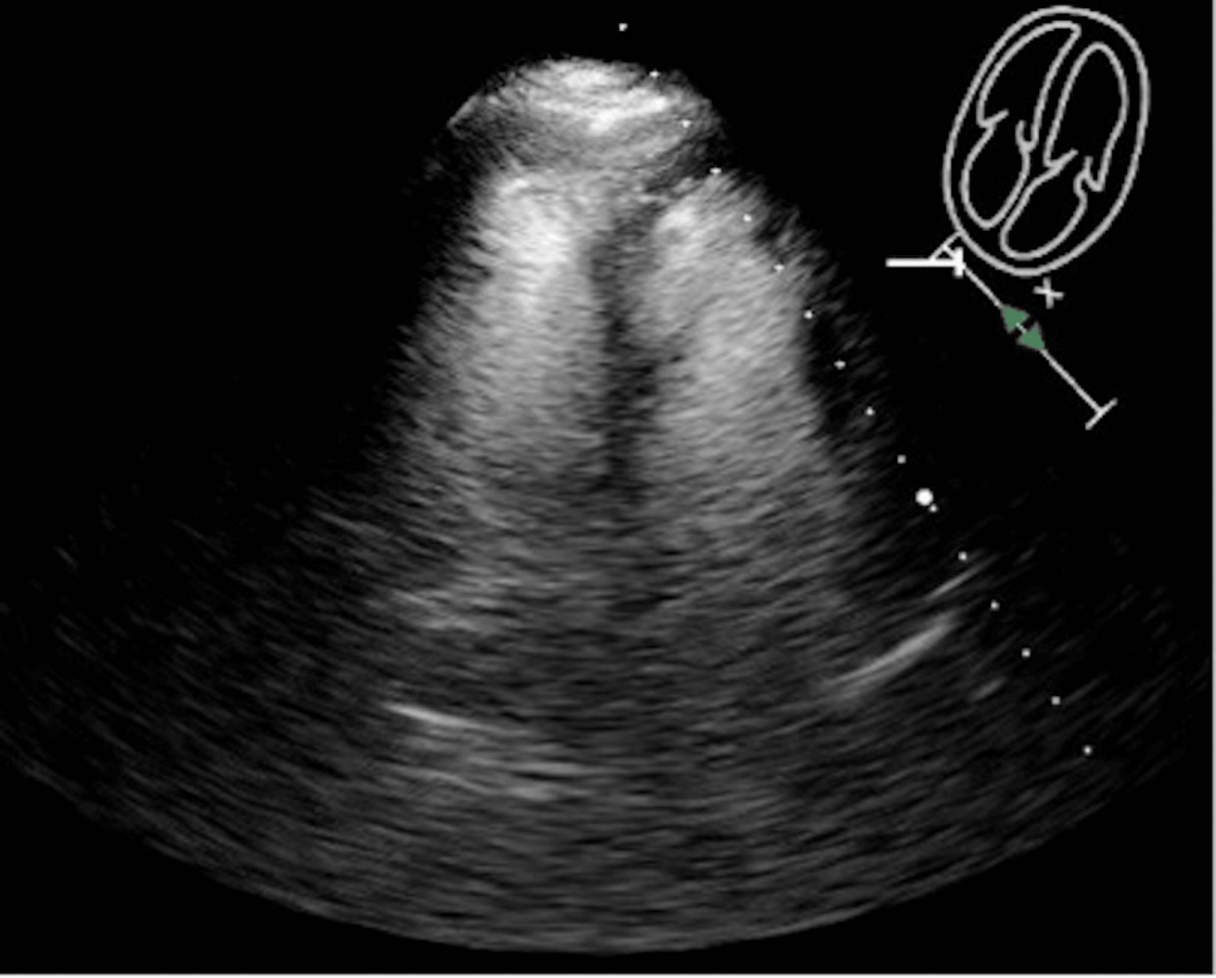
On echocardiography with contrast (four chamber view), hypokinesis of the lateral right ventricular wall and preserved apical contractility consistent with the McConnell sign is noted.
